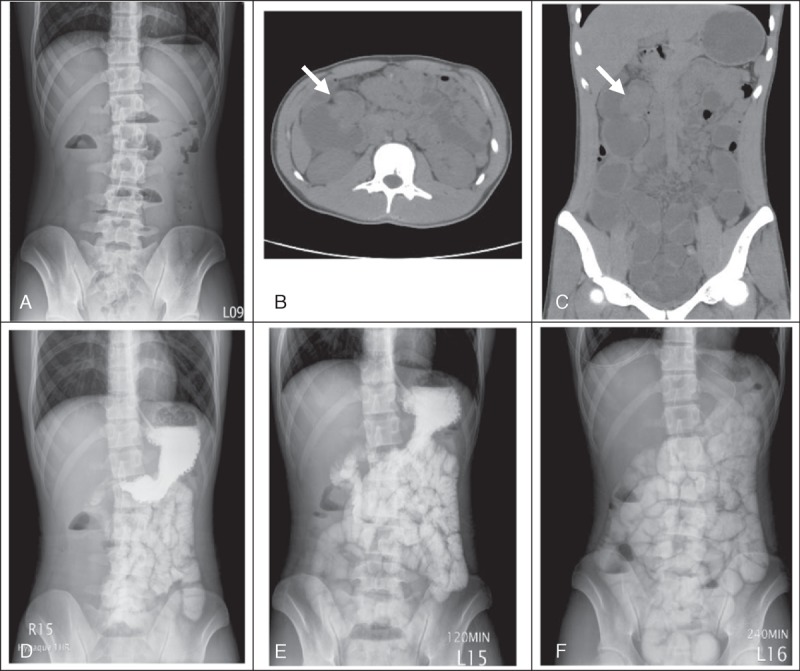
Imaging studies of the patient. (A) Plain abdominal x-ray at initial presentation. (B–C) Horizontal and coronal views of the tumor identified, retrospectively, which was initially masked by the overdistended small intestines. (D–F) Upper gastrointestinal and small bowel series on the first, second, and fourth hour after contrast ingestion, respectively.
